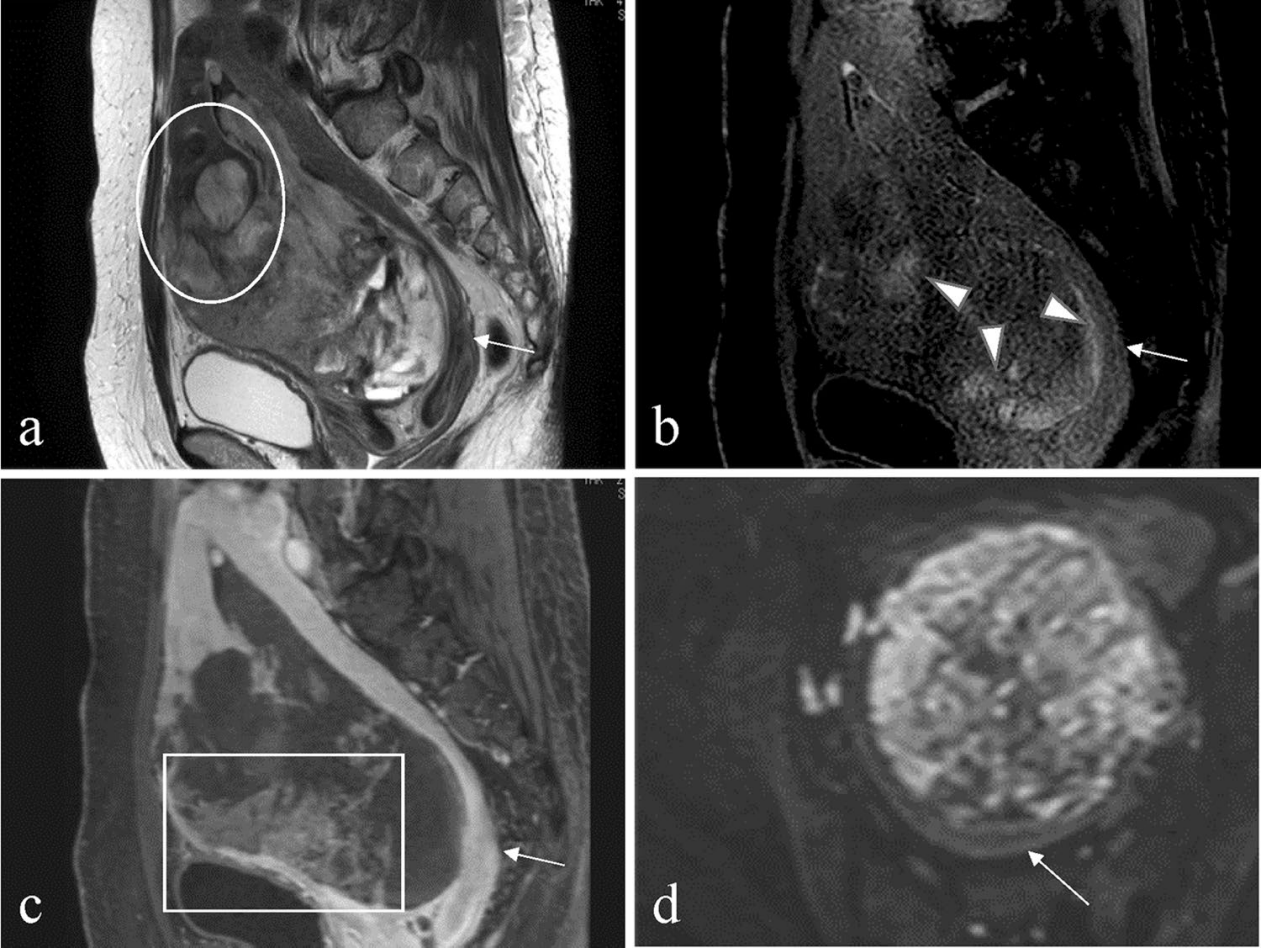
A 49-year-old woman with undifferentiated uterine sarcoma with abnormal vaginal bleeding. a The sagittal T2-weighted image reveals a tumor located mainly in the uterine cavity, with a nodular border (circle). The tumor shows a heterogeneous signal on the T2-weighted image. b The tumor shows T1 bright area (arrowhead) on the sagittal T1-weighted image. c The contrast-enhanced T1-weighted image with fat suppression indicates a lesion with feather-like enhancement (rectangle). d On an axial diffusion-weighted image with a *b*-value of 1000 s/mm^2^, the solid component of undifferentiated uterine sarcoma shows a higher signal than the myometrium (thin arrow). The lesion shows restricted diffusion, and the mean apparent diffusion coefficient value is 0.90 × 10^−3^ mm^2^/s
